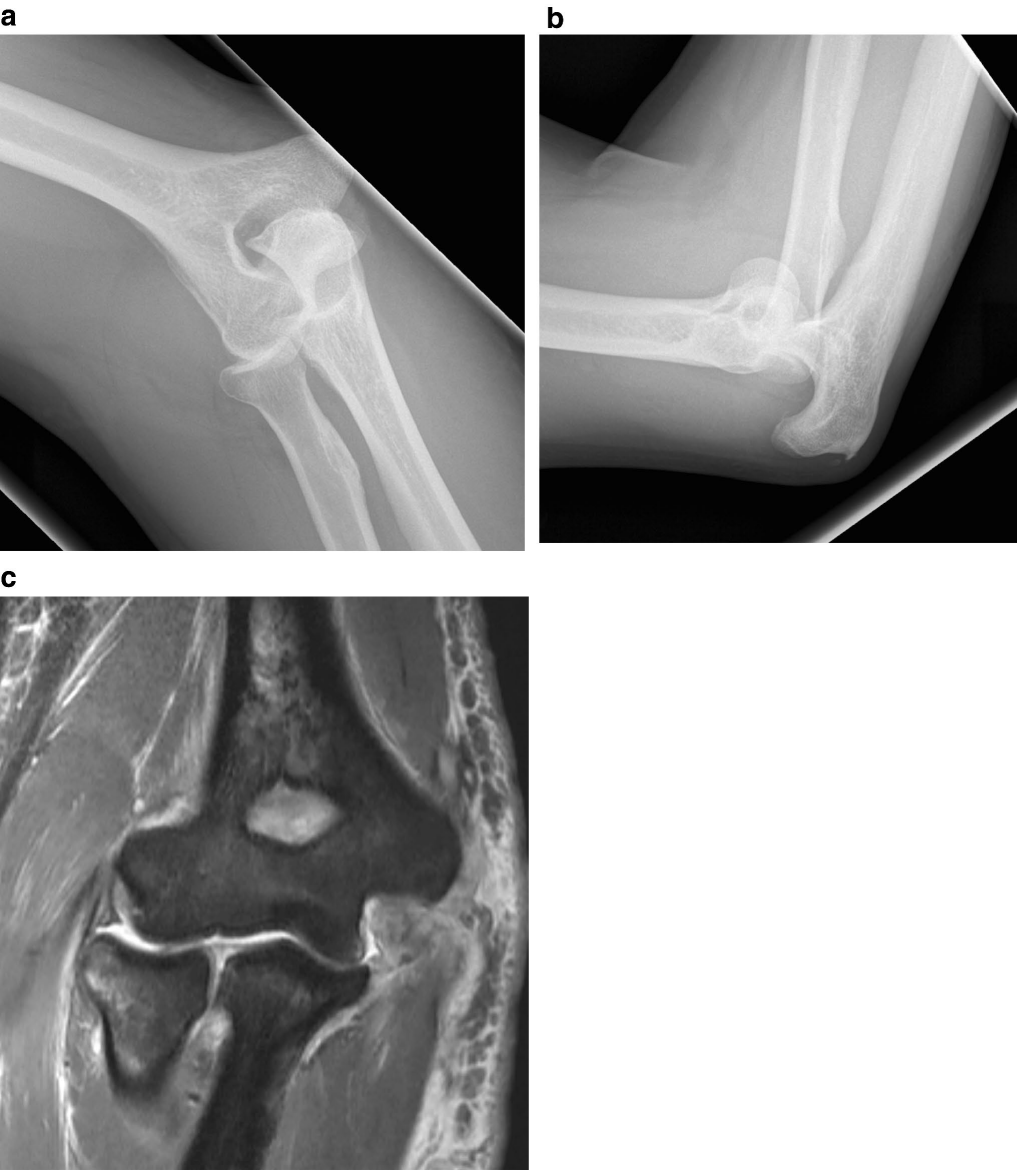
a, b Anteroposterior and lateral radiograph of a posterolateral elbow dislocation of a 39-year old patient after simple fall from standing height. c MRI was performed 7 days after injury. Coronal short tau inversion recovery (STIR) sequence shows signal abnormalities of the MCL and CFO (complete tear) as well as severe bone marrow edema laterally. The LCLC is partially detached from its humeral origin, whereas CEO are intact
